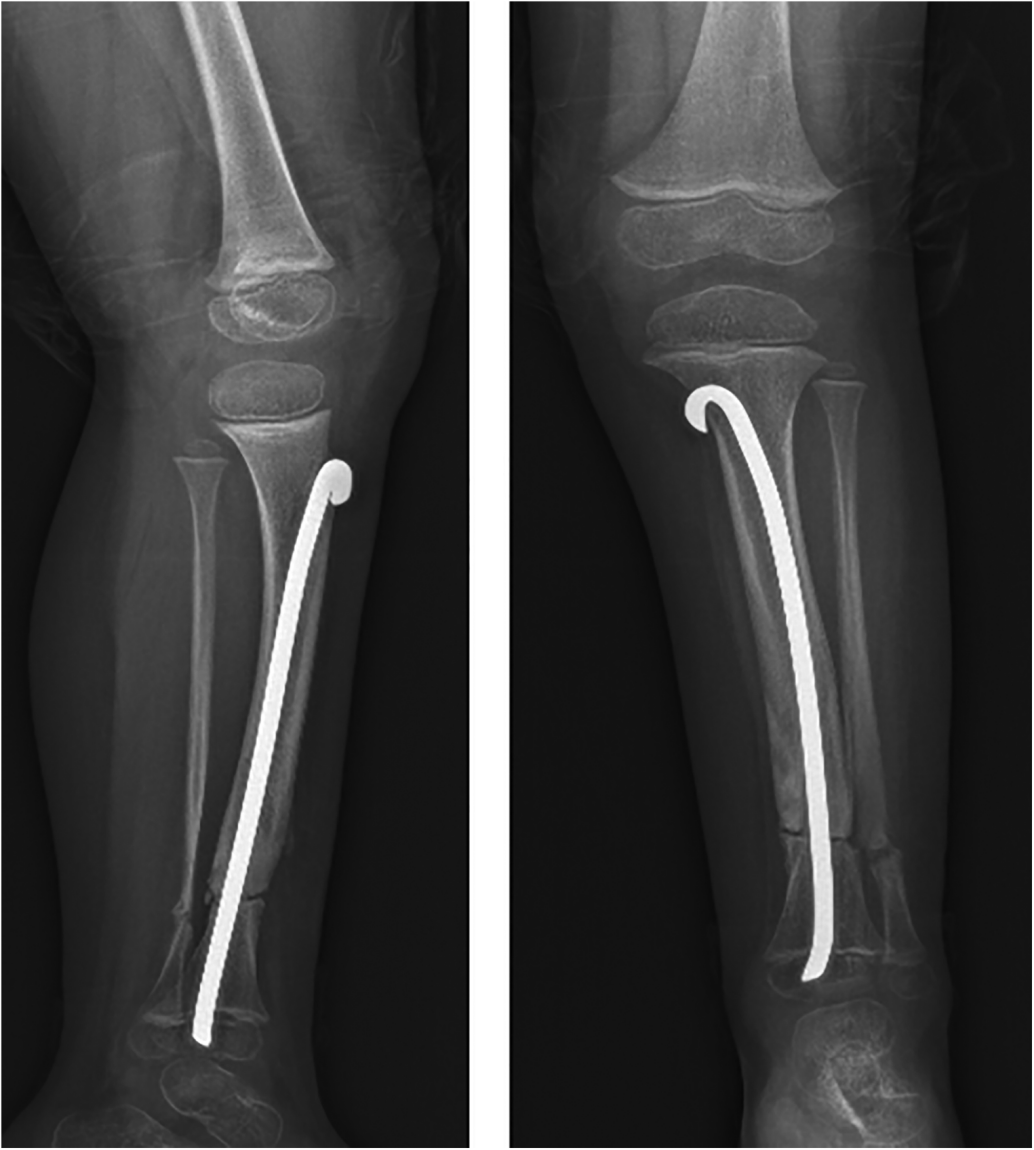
X-ray that shows the rush rod inserted across the distal tibial cartilage growth plate, preserving the ankle joint. Tibial and fibular osteotomy sites are evident.
